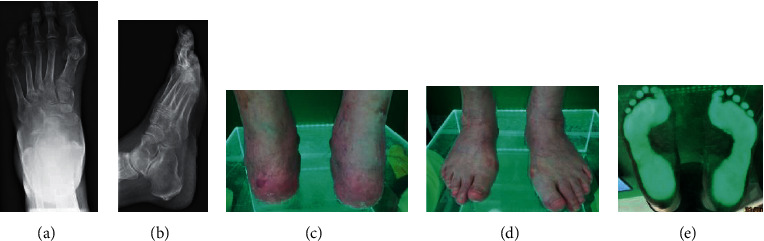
(a–e) Results of imaging tests (X-ray) and podoscopic tests: patient no. 8. (a) Dorsal-plantar X-ray: hallux valgus, subluxation of sesamoid bones, and overloaded cuneometatarsal joint. (b) Diagonal foot X-ray (lateromedial): enthesopathy of the plantar fascia and Achilles tendon, numerous calcifications, and overloads in the upper ankle joint. (c) Posterior picture: ankle joint and hindfoot evaluation. (d) Anterior picture of the feet: assessment of toe positioning and deformation. (e) Plantar photo on the podoscope: assessment of plantar part of the foot, height measurement of the longitudinal arch.
